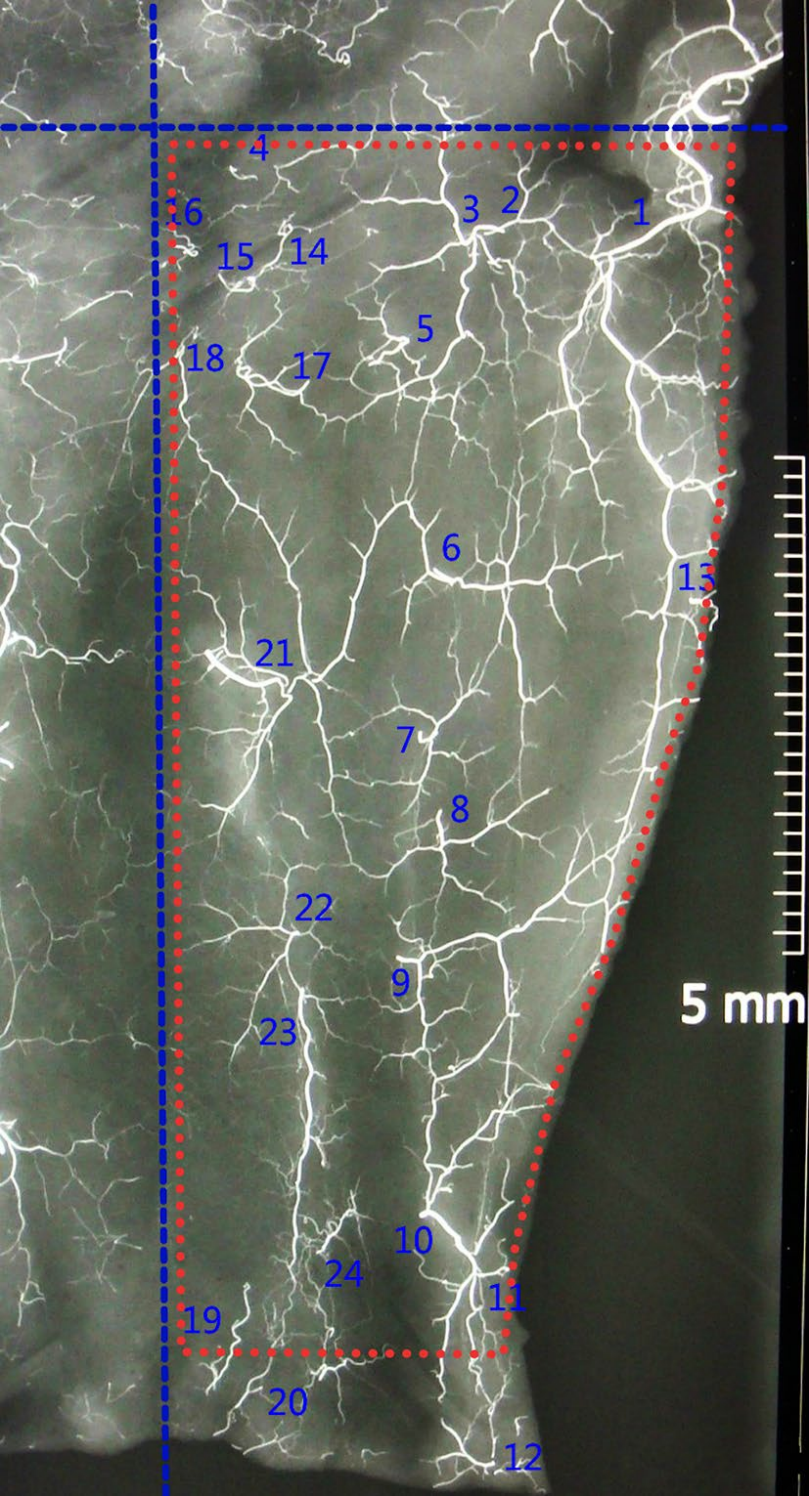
X-ray radiograph showing the arterial vasculature of the lateral leg integument. The integument was incised along the intermalleolar line and posterior midline. Transverse and longitudinal dotted lines (blue) represent the popliteal crease and anterior midline, respectively. The red dotted line displays the limit of the modified lateral gastrocnemius myocutaneous flap. The perforators arising from the inferior lateral genicular artery (2–4) were anastomosed with the perforators of the lateral popliteal cutaneous artery (1), circumflex fibular artery (5), anterior tibial recurrent artery (14, 15), anterior tibial artery (16–20), superficial peroneal artery (21, 22), and the arterial network around the superficial sural nerve (23). The perforators arising from the lateral popliteal cutaneous artery (1) were anastomosed with the perforators of the peroneal artery (6–11), lateral calcaneal artery (12) and lateral sural artery (13). The continuous arterial networks were extended up to the level of the intermalleolar line.
